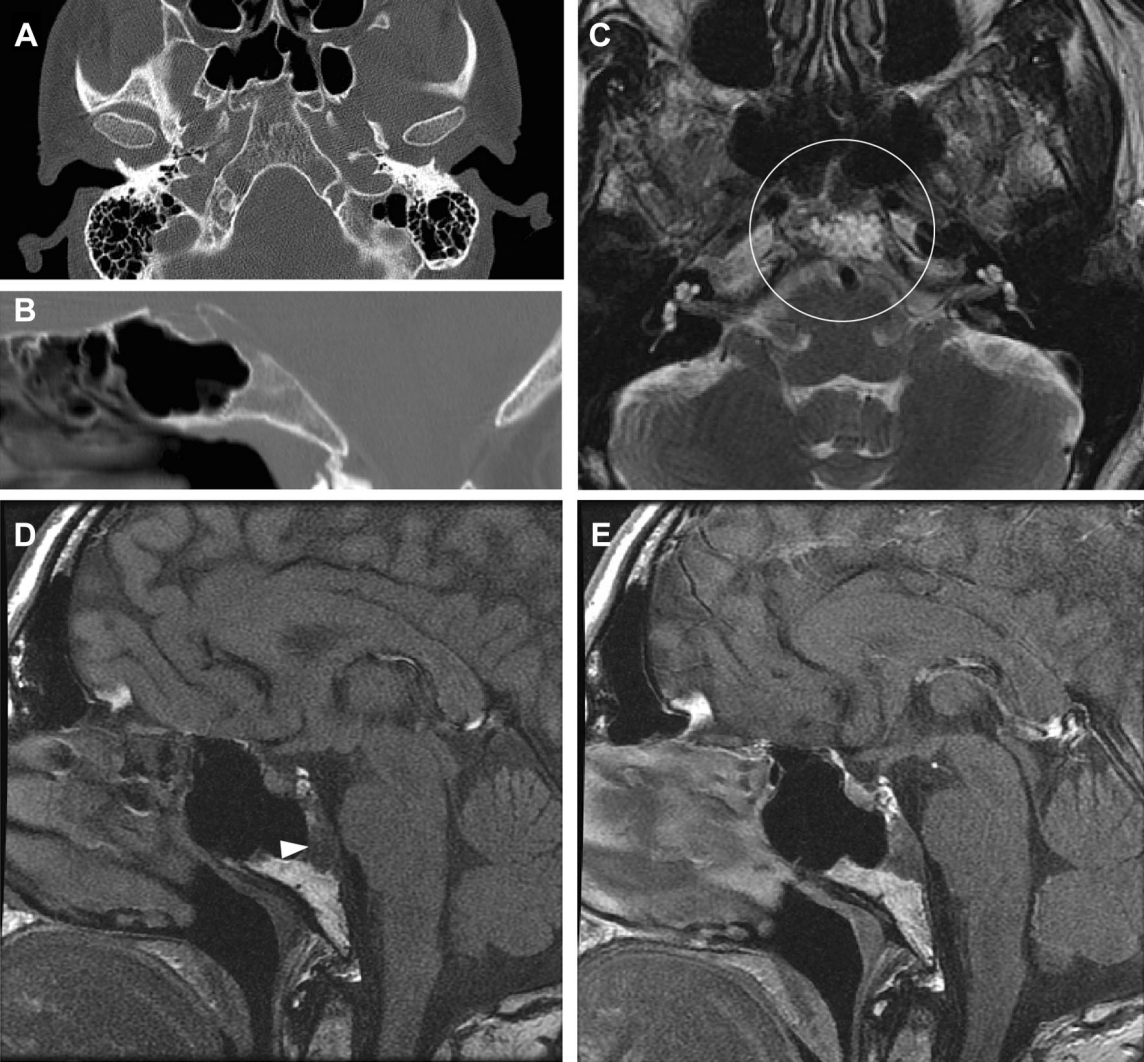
BNCT. Imaging of a well-defined intraosseous lesion in the base of the skull. CT imaging of the clivus in axial (a) and sagittal (b) plane shows normal bone trabeculation. There is no trabecular or cortical destruction. T2-weighted MRI imaging in axial plane (c) shows a hyperintense mass at the clivus, which is hypointense on T1-weighted imaging (arrow, d) and does not show contrast enhancement (e), as opposed to the physiological contrast enhancement of the pituitary gland. This tumour shows typical imaging characteristics of a benign notochordal cell tumour. However, there was no pathologic confirmation. On follow-up MRI after 1 year, the lesion was unchanged
